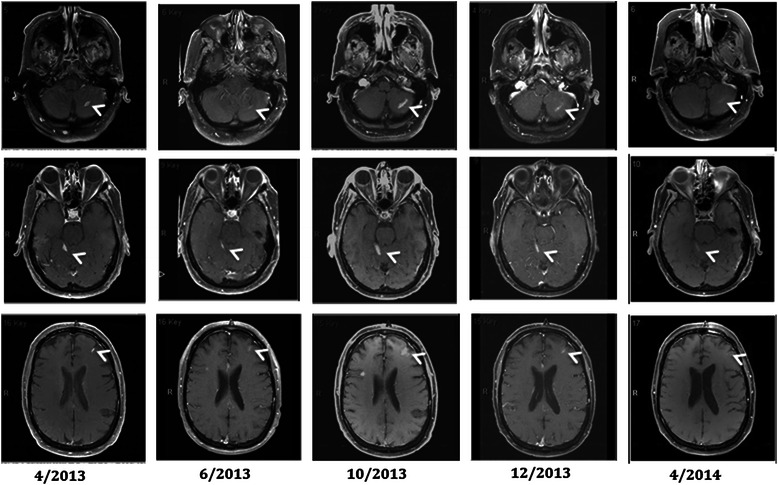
Brain MRI showing the response and the progression of the leptomeningeal disease with BRAF inhibitor-based therapy. The arrows indicate the enhancement of the leptomeninges in April 2013. After vemurafenib therapy, the follow-up images revealed initial regression of the leptomeningeal disease. He had progression of the leptomeningeal disease in October 2013 and started whole brain radiation followed by dabrafenib plus trametinib. The follow-up scans showed regressed leptomeningeal disease
